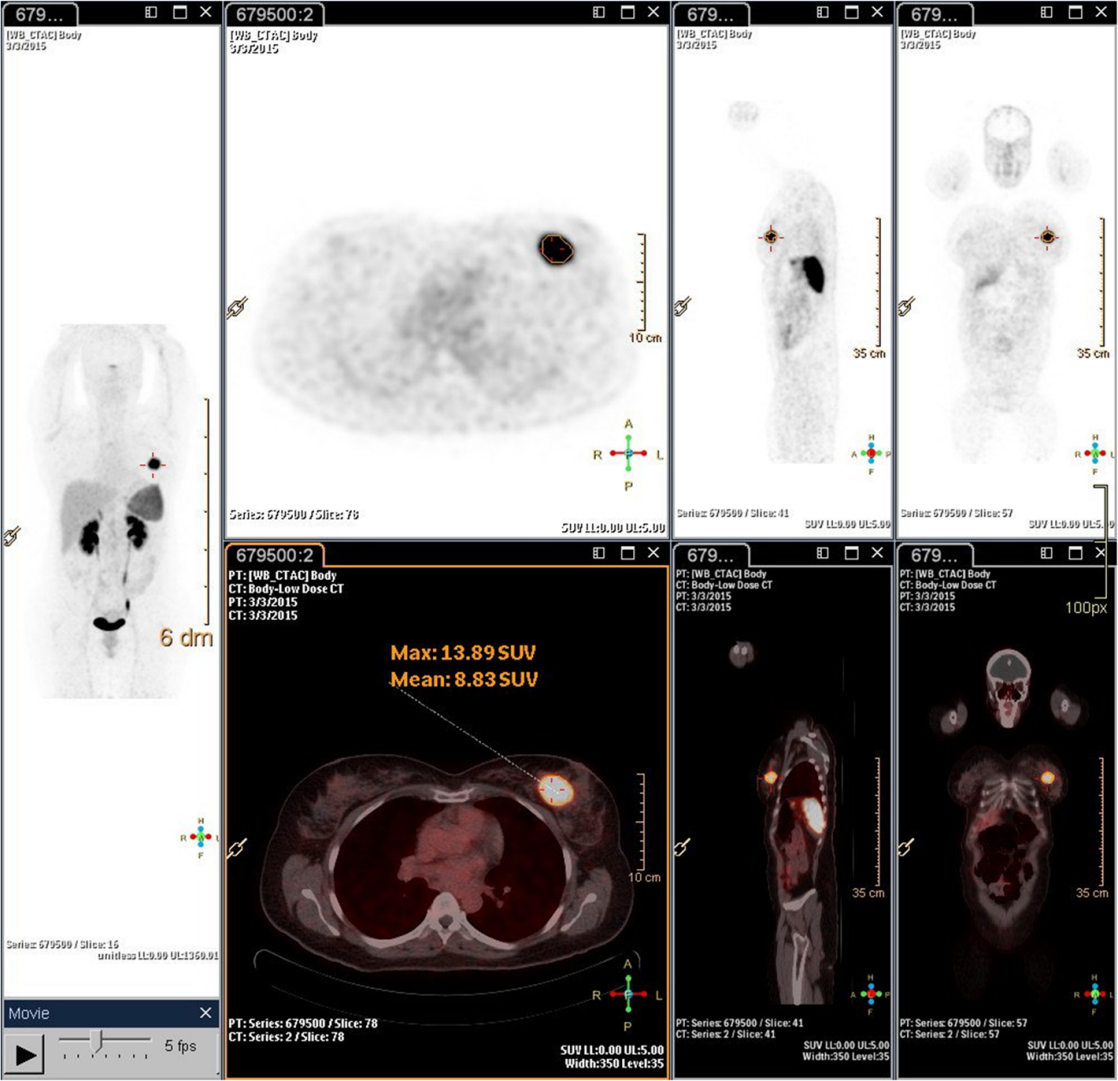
Positron emission tomography-computed tomography scan fused images for the whole body, showing the primary breast lesion and no extra-mammary involvement
